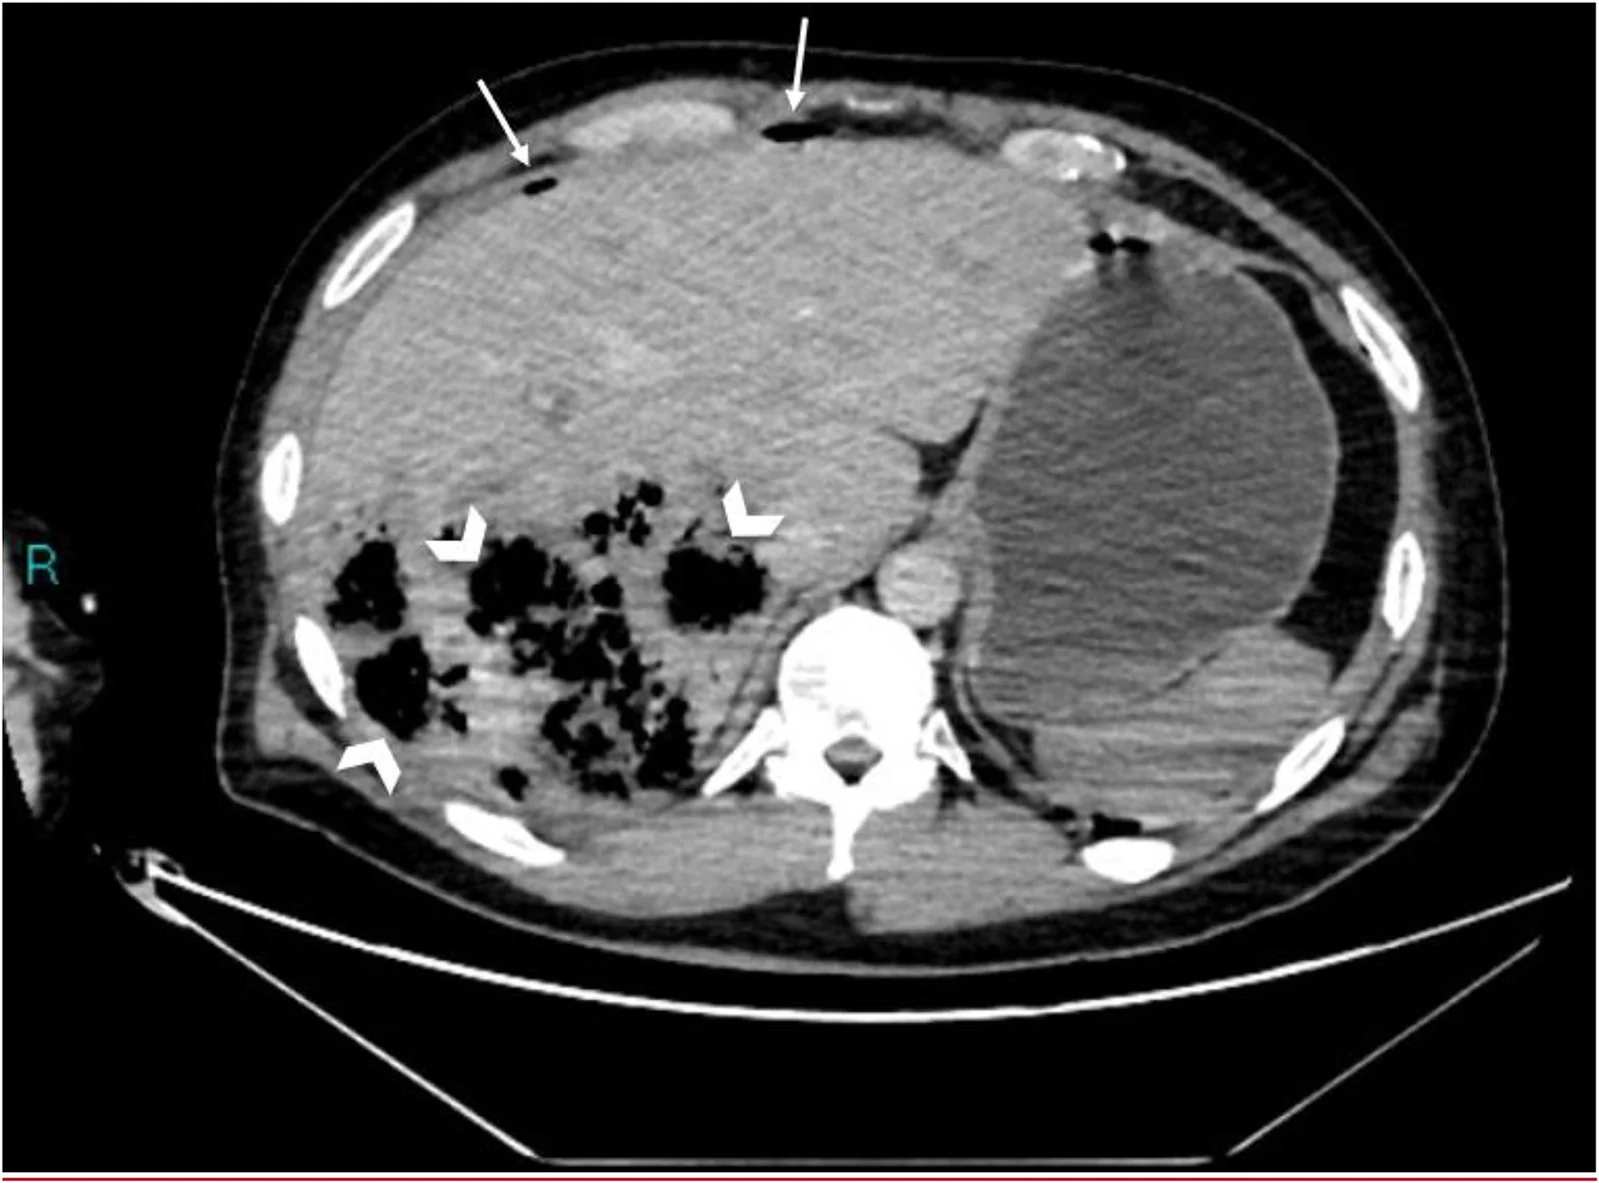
Axial CT section through the superior aspect of the liver. There is a small volume of pneumoperitoneum anterior to the liver indicating breach of the liver capsule (white arrows). Multiple gas-filled areas in segment VII and VIII of the liver (white arrow heads) without wall enhancement.
